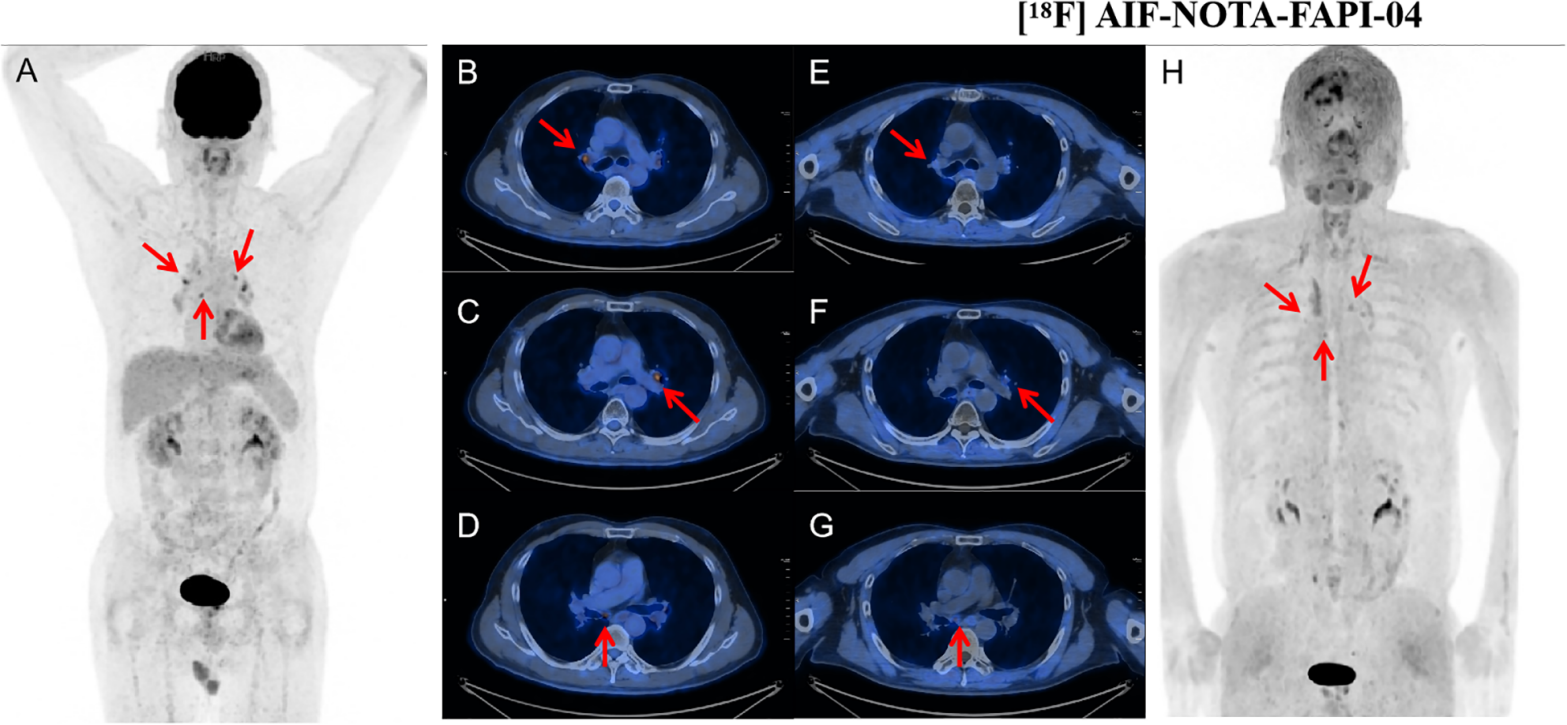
A 51-year-old man with lung adenocarcinoma who had undergone right superior lobe wedge resection 1 year ago. [^18^F]FDG PET/CT showed multiple lymph nodes in bilateral hilar (fused PET/CT: B, C, E, and F, arrow) and subcarinal zone (fused PET/CT: D and G, arrow) with high uptake and SUVmax of 6.3–7.0. [^18^F]AlF-NOTA-FAPI-04 PET/CT showed low-to-moderate uptake of lymph nodes at corresponding sites, SUVmax 2.5–3.6. Subsequent imaging showed no significant changes in the lymph nodes in the corresponding area, confirming that these lymph nodes were benign. [^18^F]FDG PET/CT images (A–D) and [^18^F]AlF-NOTA-FAPI-04 PET/CT images (E–H): maximum-intensity projections (A, H). SUVmax, maximum standard uptake value.
